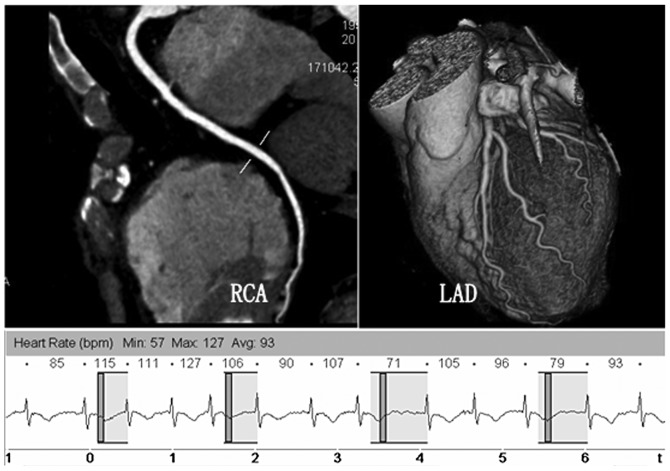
Female, 58-year-old patient, BMI=20.69 kg/m^2^. The HRV was 44 bpm during scanning. MHR and heart rate range were 93 and 57–127 bpm, respectively. Image quality score was 5 points. CT coronary angiogram (left) and volume-rendered reconstruction (right) show high quality images of the RCA and LAD. BMI, body mass index; HRV, heart rate variability; MHR, mean heart rate; RCA, right coronary artery; LAD, left anterior artery.
